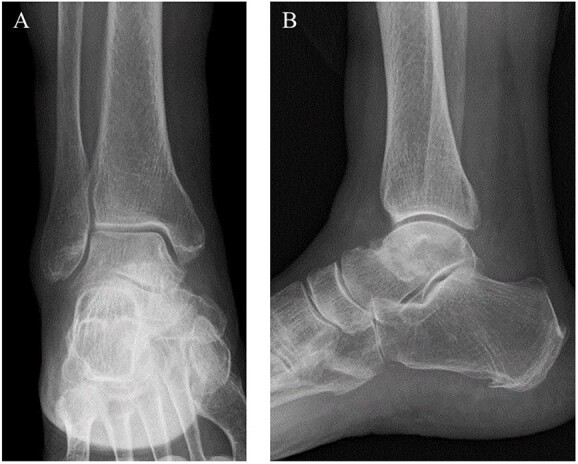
Preoperative plain radiographs showed no obvious abnormal findings from the (A) anteroposterior and (B) lateral views.
